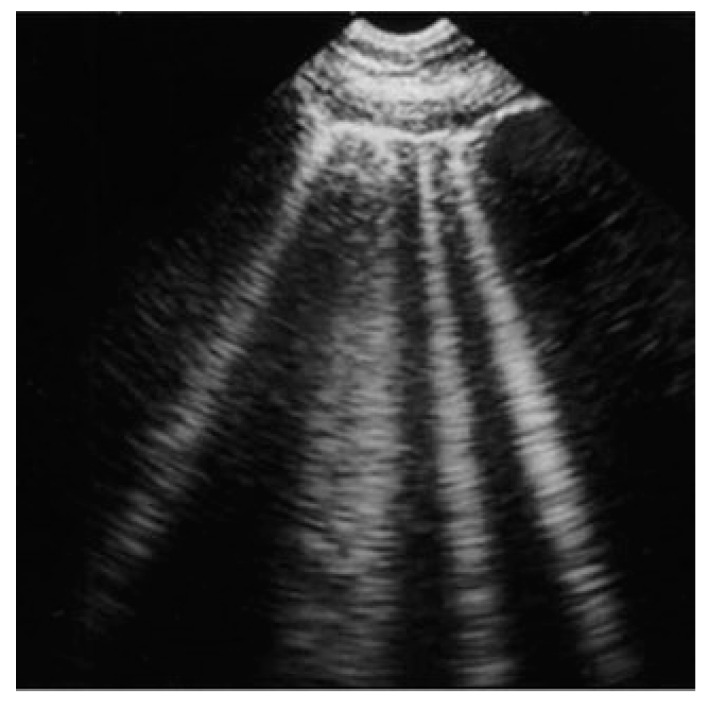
B-lines in lung ultrasound scan. B-lines, or ultrasound lung comets, can be visualized as discrete, laser-like, vertical hyperechoic reverberation artifacts, originating from the pleural line and extending to the image depth without intensity reduction. They move synchronously with lung sliding.
